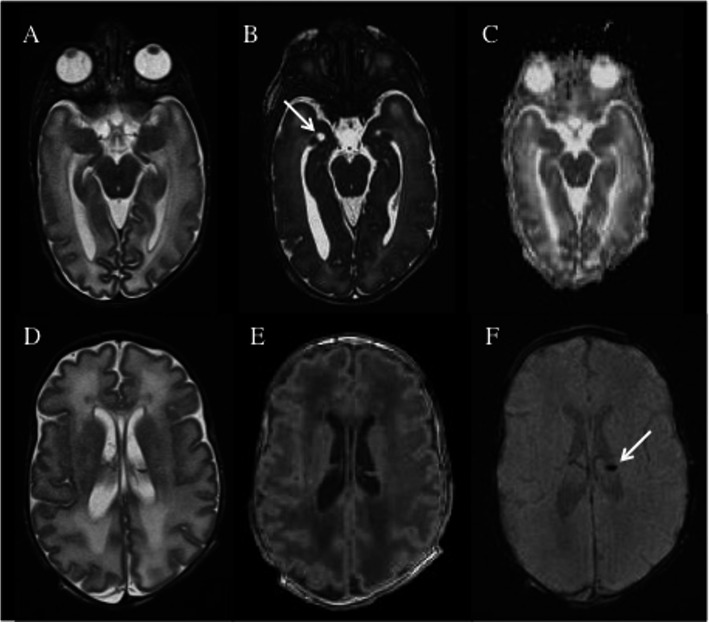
MR scan show an immature aspect of the gyration of the cerebral hemispheres associated with a reduced myelination (A and D, axial T2-weighted images; C, axial Diffusion Weighted Imaging DWI; E, volumetric axial-reformatted T1 weighted image). T2-weighted sections (A and D) revealed increased hyperintensity of the parieto-occipital and temporal white matter with cysts in the bilateral periventricular temporal region (white arrow in B, True Fast Imaging with steady state procession (TrueFISP) axial isotropic volumetric T2 weighted image) . Germinolytic cysts with hemosiderin deposit were depicted in the thalamocaudal notch region bilaterally (white arrow in F, axial Susceptibility Weighted Imaging (SWI))
